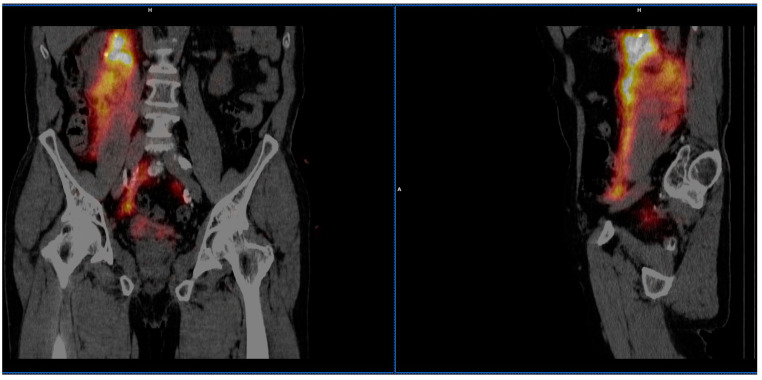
SPECT/CT example of no lymphatic outflow and radiotracer spillage after radiotracer injection performed at the site of the tumor located in the renal pelvis.
